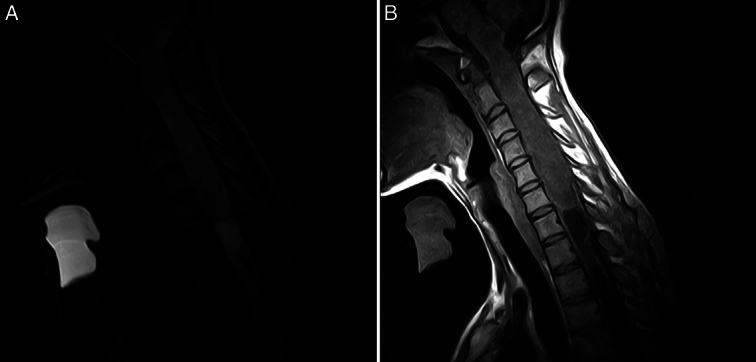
(Colored). A. MRI, sagittal section, T2: Shows a heterogeneous hyperintense lesion extending from C7 to the medulla. B. MRI, sagittal section, T1: Shows a heterogeneous Isointense lesion extending from C7 to the medulla with a hypointensity extending caudal to the mass (Syrinx).
